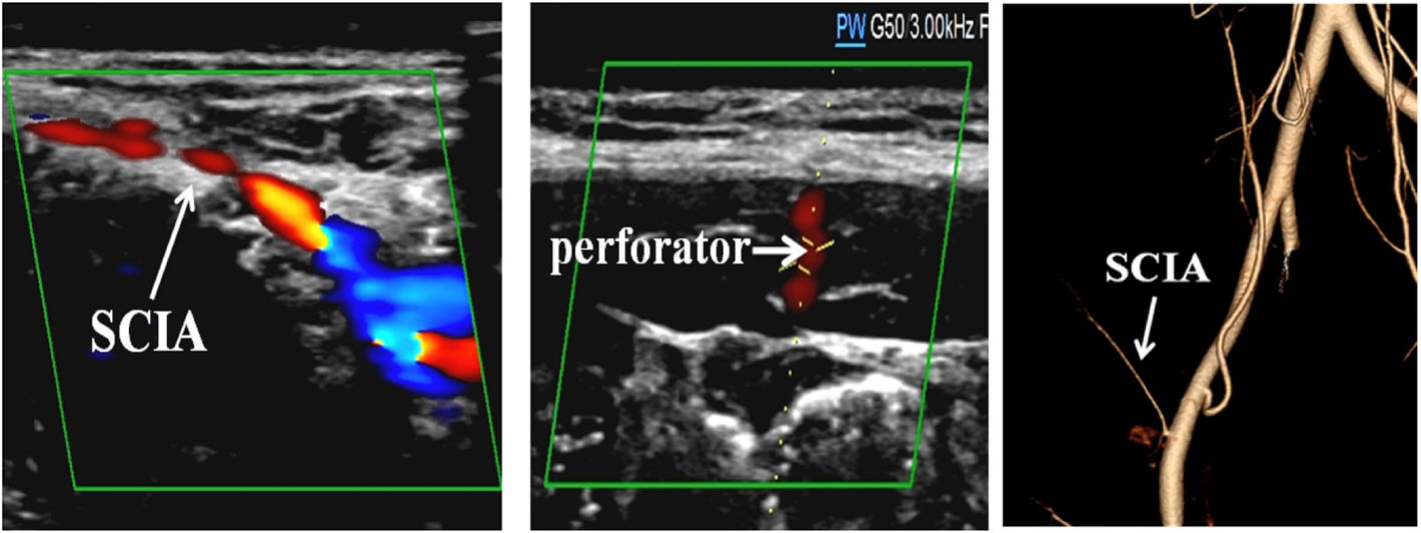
Preoperative CTA and CDS examinations
